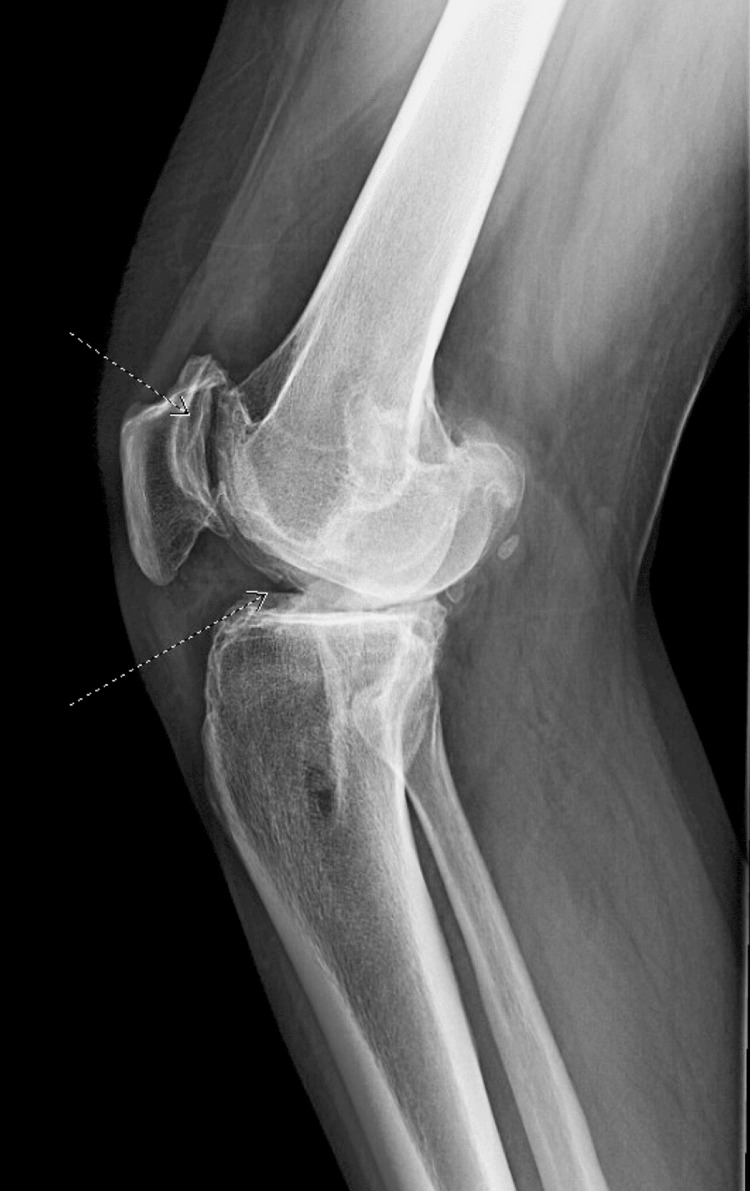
Preoperative radiographs. Lateral view of right knee, showing degenerative changes. Arrows show diffuse joint space narrowing and osteophytes.
